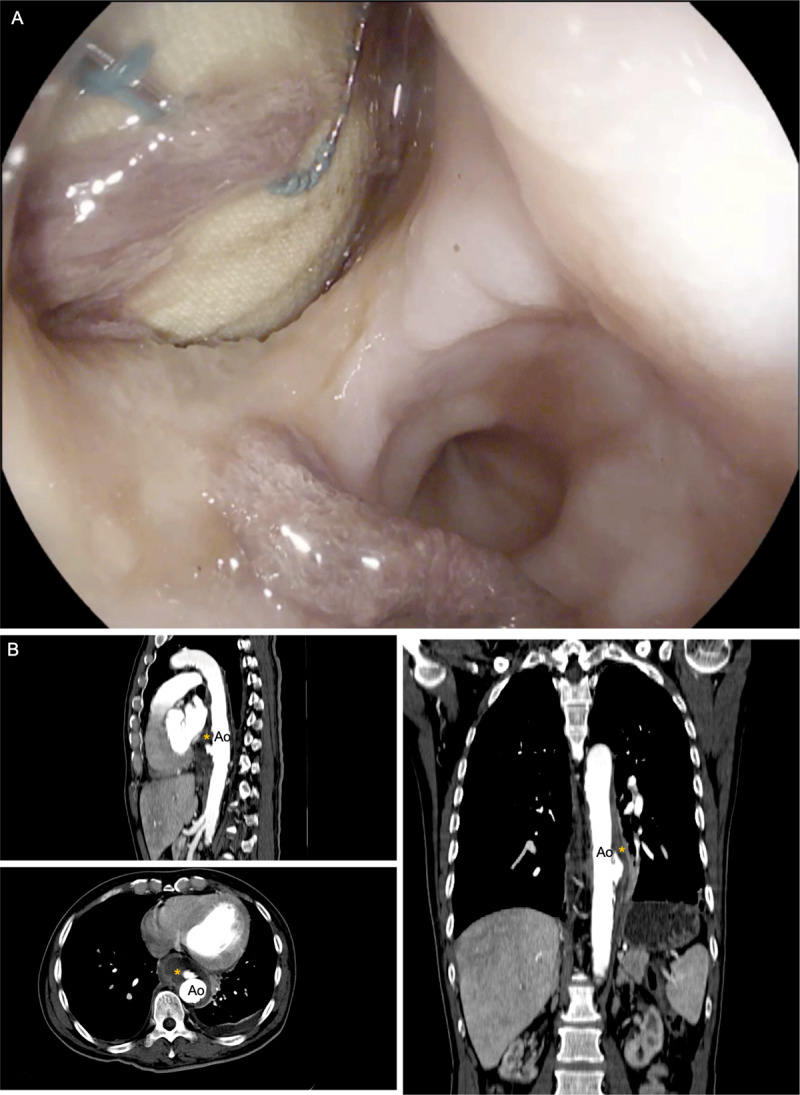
Aortoesophageal fistula. (A) Esophago-gastro-duodenoscopy. (B) Computed tomographic angiography. Ao: descending thoracic aorta; *: esophagus.
